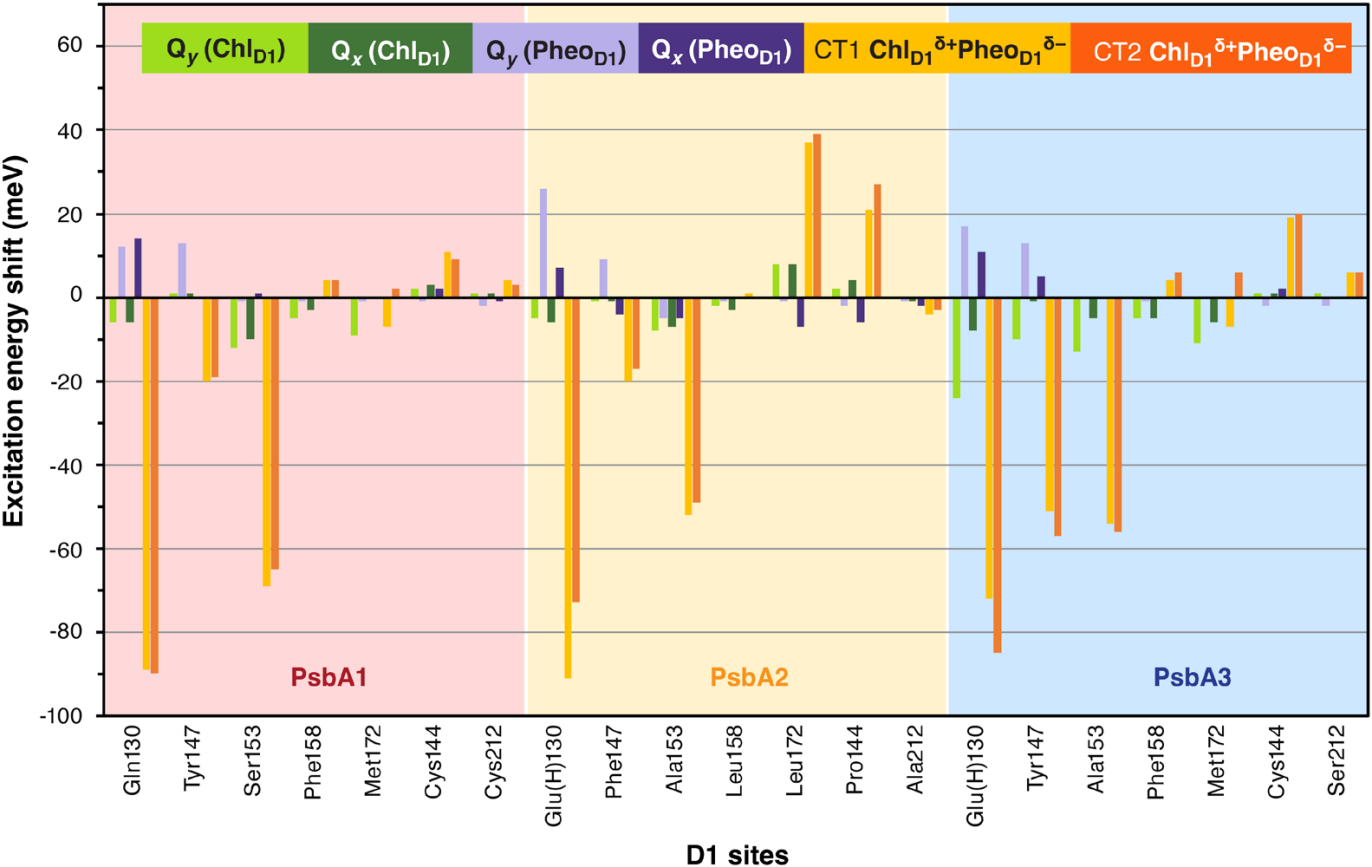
Electrostatic contributions of selected D1 residues within PsbA1, PsbA2, and PsbA3 proteins to the overall electrochromic shifts of the first two local excitations and the two lowest CT states of the Chl_D1_–Pheo_D1_ pair. Negative values indicate a red-shift and positive values indicate a blue-shift. Complete data are provided in *SI Appendix*, Table S8.
